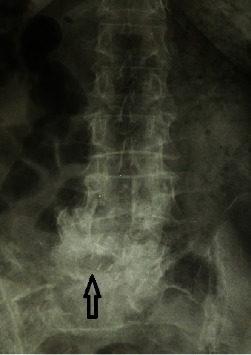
Preoperative anteroposterior roentgenogram of the patient. The arrow shows a previously inserted intervertebral PEEK cage.
